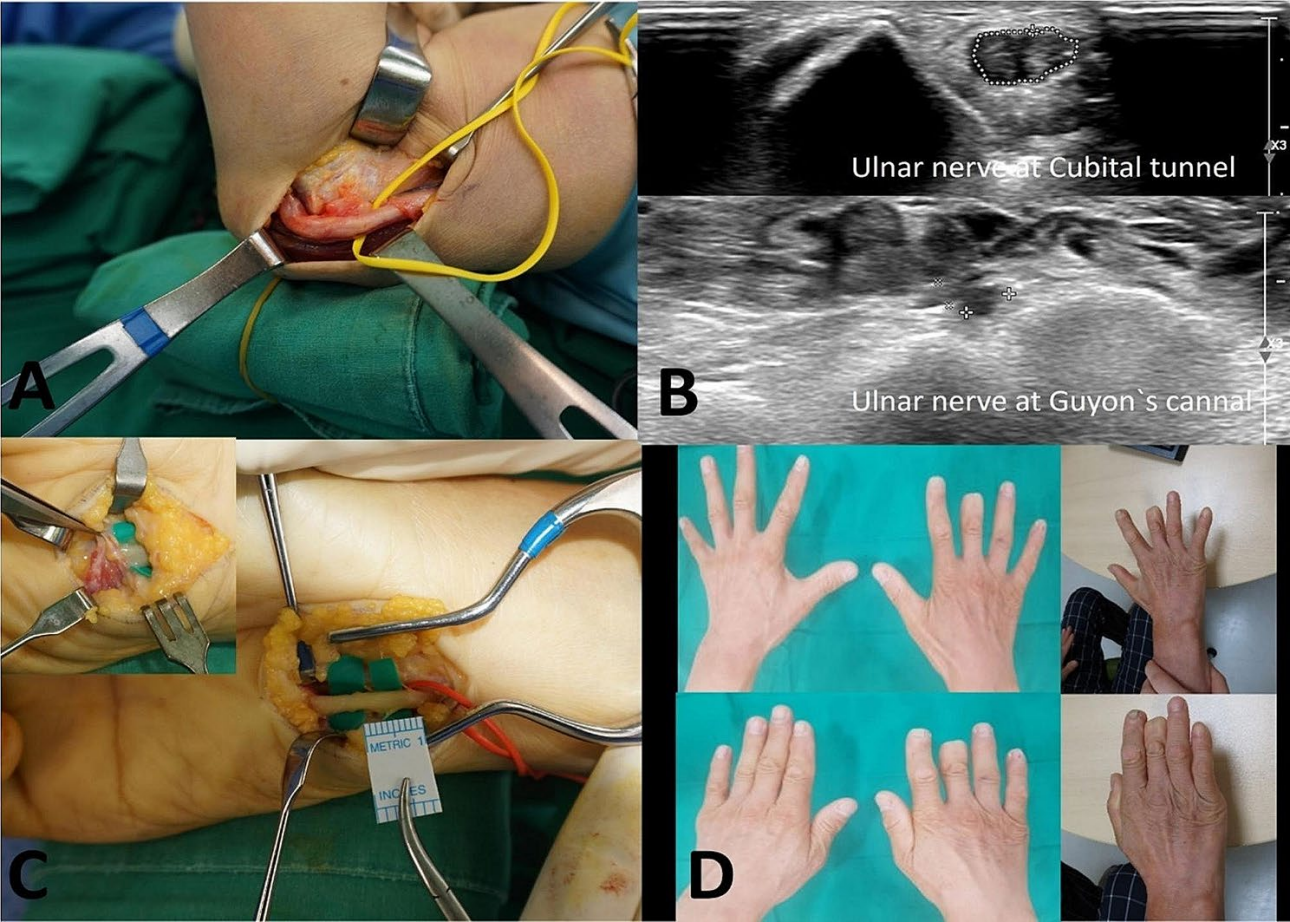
A sixty-one years old male came for right 4,5th finger tingling sensation and 1st webspace atrophy of right hand. Double crush syndrome was confirmed by EMG/NCS with Cubital tunnel syndrome and Guyon’s canal syndrome. (A) Intraoperative exploration, the ulnar nerve was compressed with swelling at the right elbow, with nerve dislocation upon flexion the elbow to 90 degrees. (B) The preoperative ultrasonography of right elbow view shows segmental swelling of ulnar nerve in cubital tunnel and ultrasonography of right wrist view shows a thicker deep branch of the ulnar nerve in Guyon’s canal. (C) Intraoperative exploration, the ulnar nerve was compressed with swelling exerted by the short palmaris brevis muscle at the right wrist. (D) On physical examination of last follow up, 1st web space atrophy was improved. (Mcgowan stage 0)
